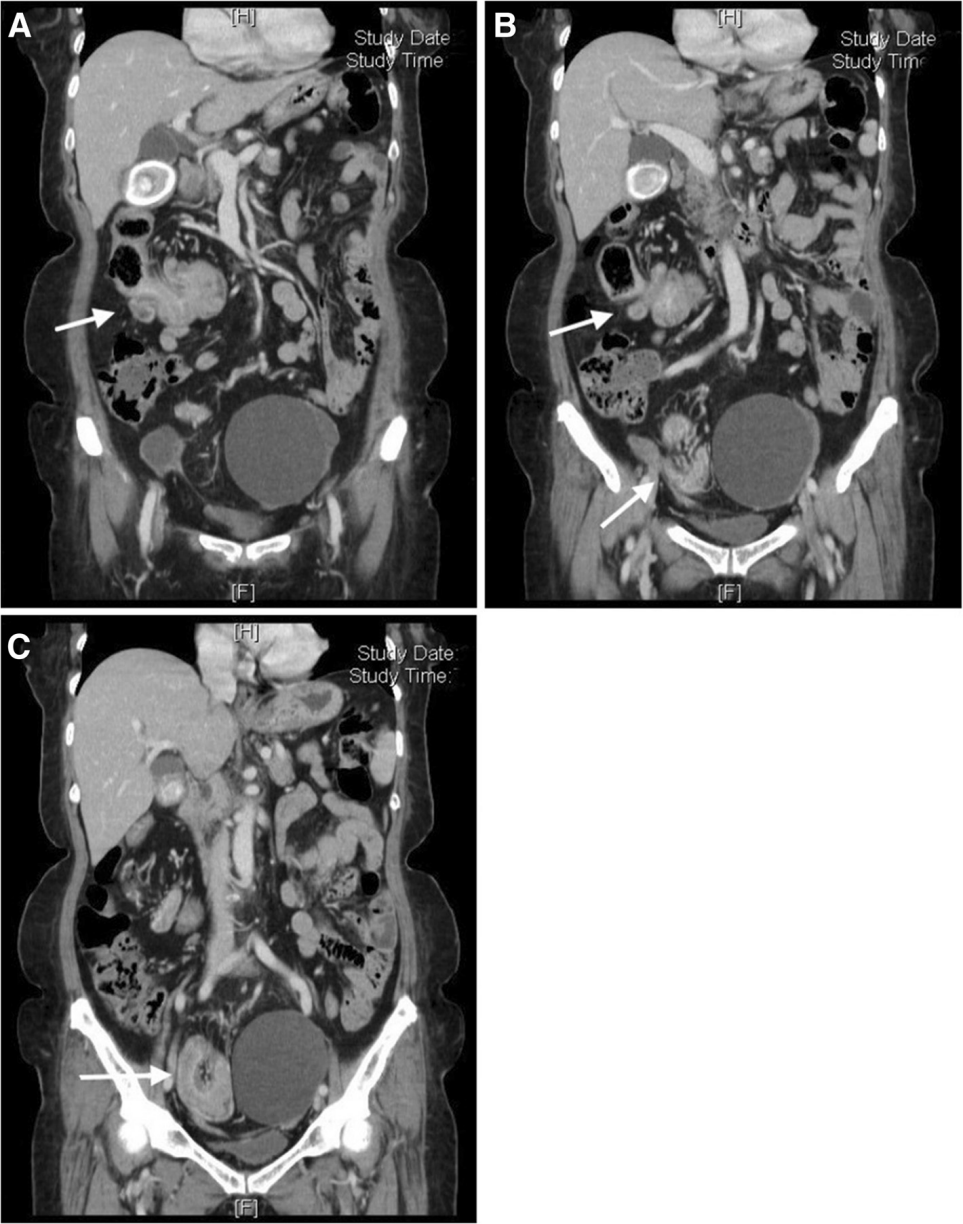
Computer tomography (CT) (arrows) showed the characteristic signs of the edematous bowel wall and mesentery within the lumen at the proximal transverse colon (A and B) and the rectosigmoid junction (B and C).
